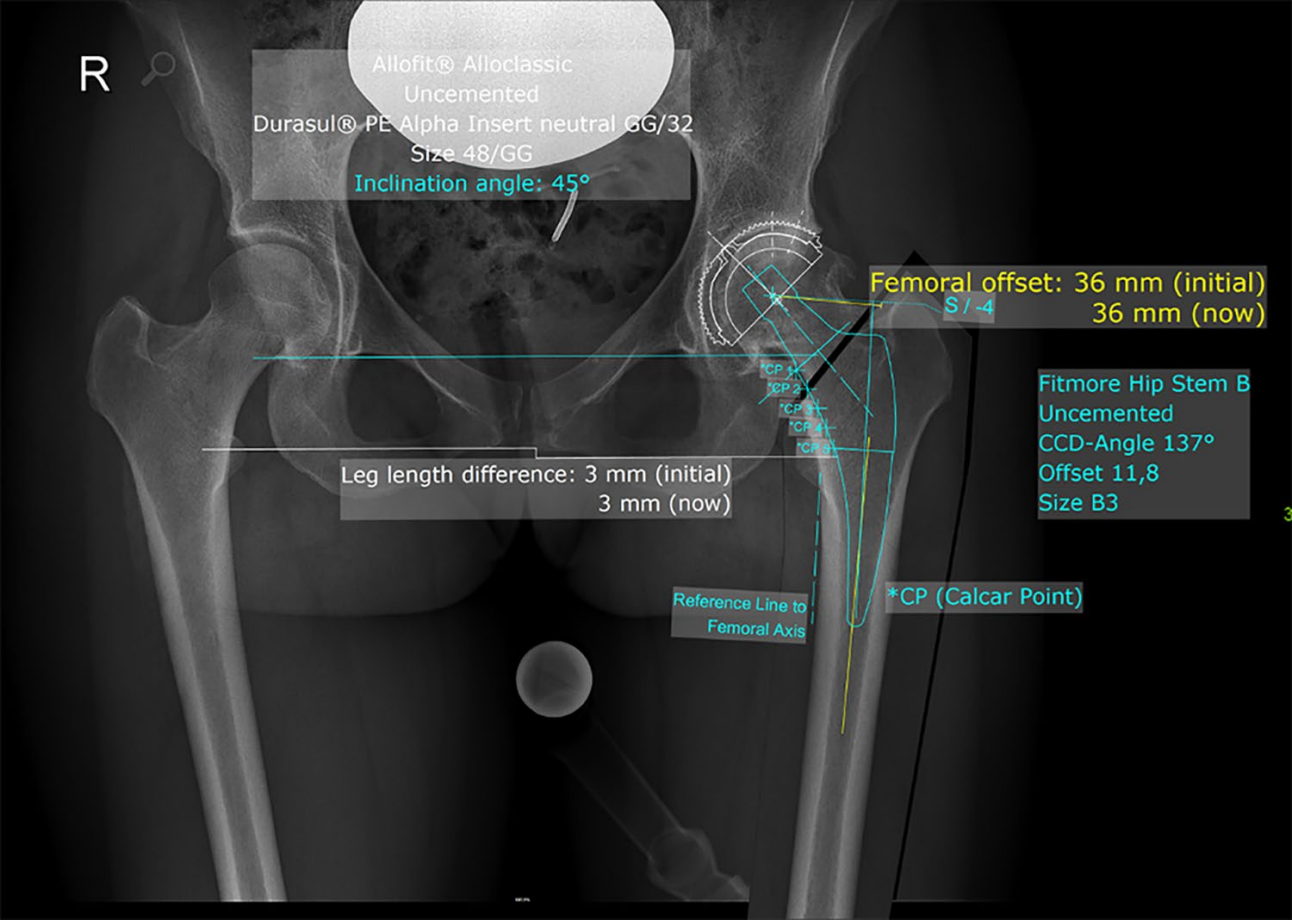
Preoperative digital template on an anterior–posterior radiograph of the pelvis
